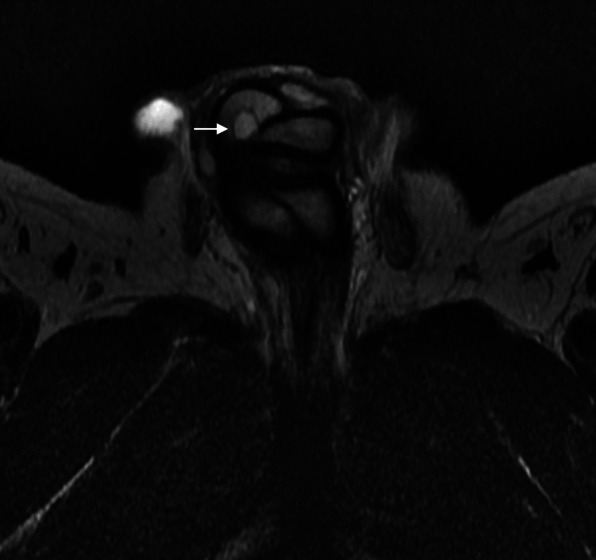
Axial T2-weighted MRI of penis and nodule. Axial T2-weighted MRI of hyperintense nodule (arrow) is seen within the right corpus cavernosa
